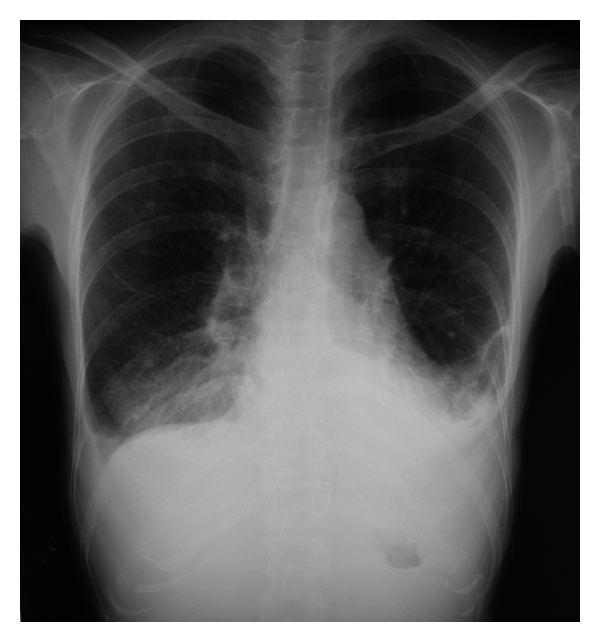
Chest X-ray showing pleural effusion 53 days postburn.
